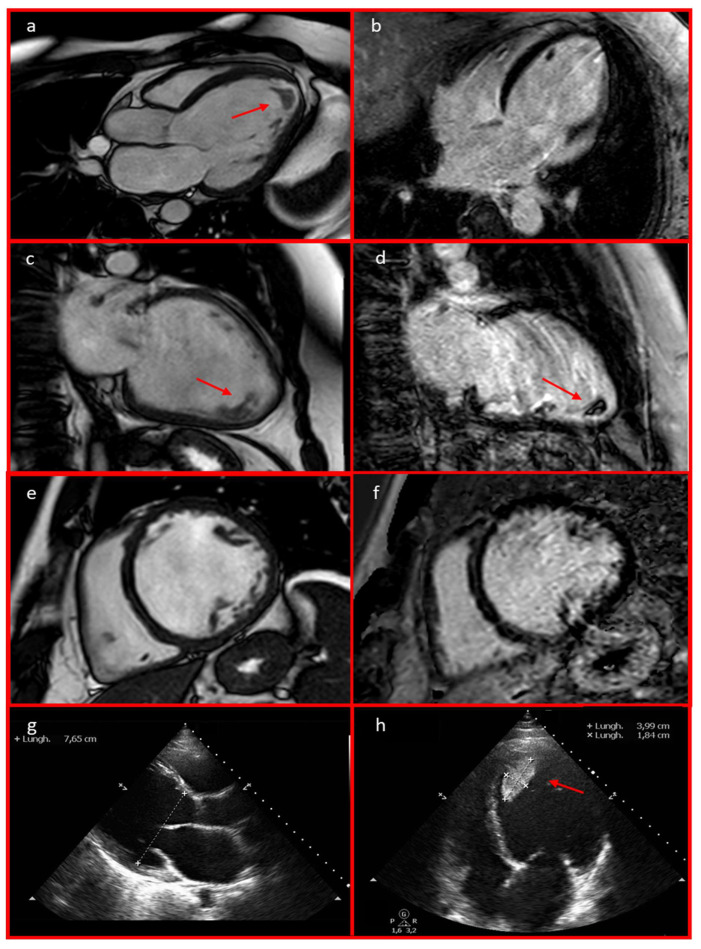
Cardiac MRI 3-chamber (a), 2-chamber (c), and short-axis (e) views revealed left ventricular dilation with severe biventricular dysfunction. These findings were also observed on the echocardiography in parasternal long-axis (g) and 4-chamber (h) views. An apical thrombus with a mobile component measuring 39.9 × 18.4 mm was identified (a,c,d,h; red arrows). Cardiac MRI late gadolinium enhancement (LGE) long-axis 4-chamber (b), 2-chamber (d), and short-axis (f) views excluded the presence of LGE.
